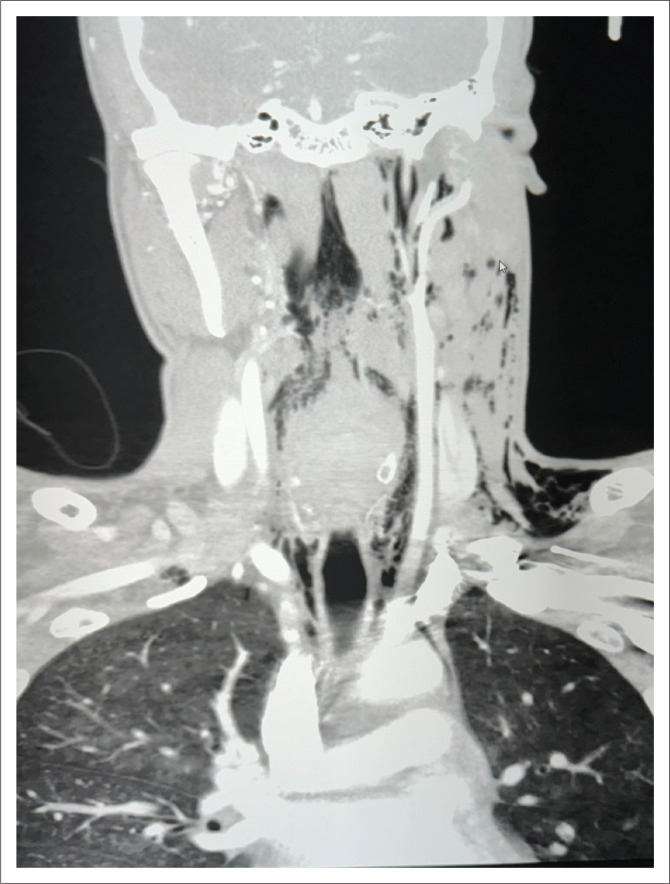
Coronal CT scan image of the neck and upper chest demonstrating superficial and deep surgical emphysema post penetrating neck trauma, suggestive of oesophageal injury.
